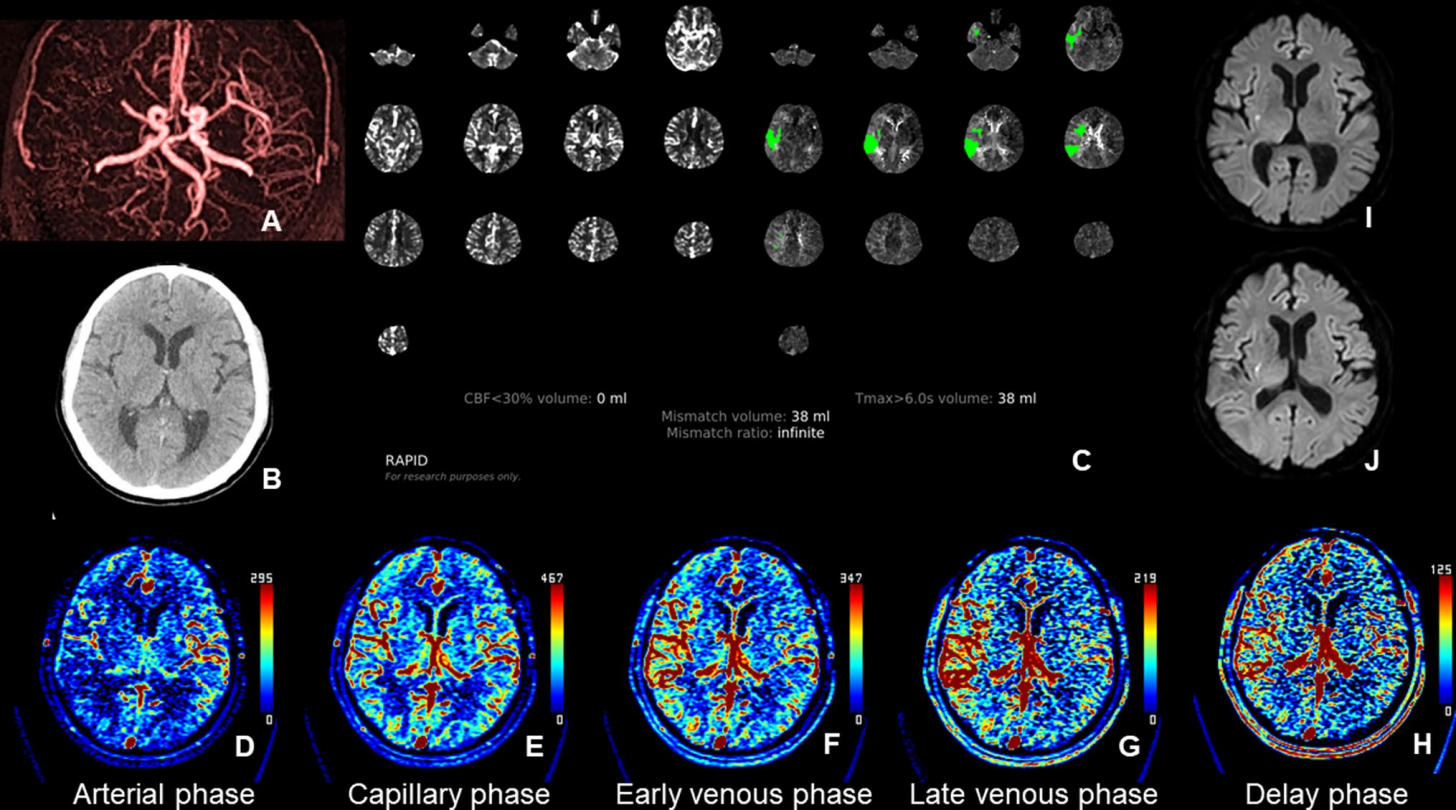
Images of a 76-year-old man with occlusion of the right middle cerebral artery (MCA) demonstrated on CT angiography (A) derived from CT perfusion (CTP). The patient had a premorbid modified Rankin Scale (mRS) score of 0 and an National Institutes of Health Stroke Scale (NIHSS) score of 3 at admission. He was managed with conservative treatment, and his 90-day mRS score was 2. The brain CT scan at admission (B) showed no abnormal findings. CT perfusion analysis using RAPID software (RAPID, RapidAI®, Menlo Park, CA, USA) (C) revealed a large penumbra, indicating potential for infarct growth. However, the CT collateral map (D–H) derived from CTP at admission displayed excellent collateral perfusion (collateral perfusion score of 5: no or minimal collateral perfusion delay in the ischemic MCA territory during the capillary phase), suggesting minimal risk of infarct progression. A small acute infarct signal was observed in the right striatocapsular region on the admission DWI (I), and the baseline lesion showed no significant growth on DWI performed on Day 1 (J)
